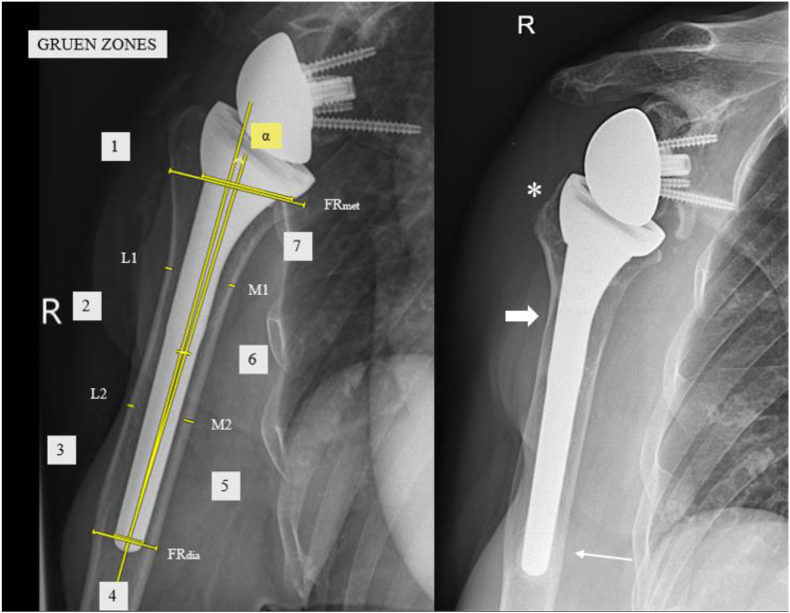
(*Left*) Overview of measurements on radiographs. Gruen zones are depicted in gray squares. Alpha angle (α) is measured between the anatomical axis of the humeral shaft and the long axis of the humeral stem. Filling ratio (FR) at the level of the diaphysis and metaphysis is defined by the quotient of the stem diameter and the humeral diameter at the appropriate level. (*Right*) Follow-up radiograph after 12 years. Asterisk shows greater tuberosity resorption. Big arrow points towards cortical thinning (most pronounced at the level of M1 in this case). Small arrow shows pedestal formation at zone 4.
